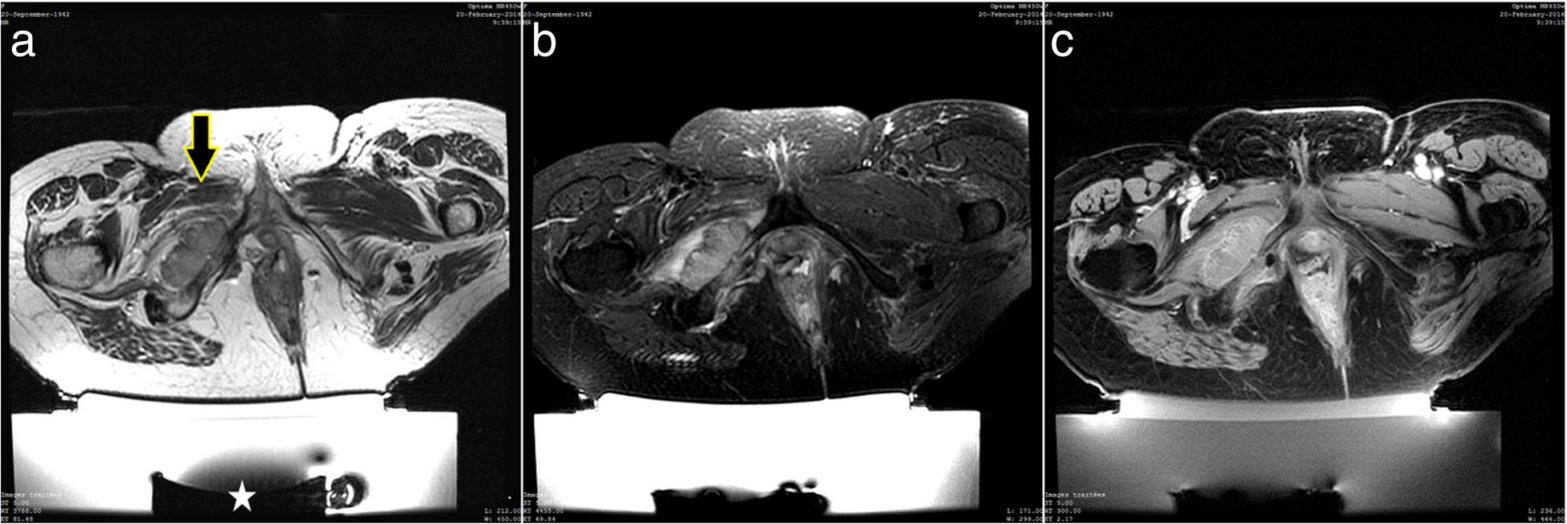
Patient 5. a Axial T2-weighted MR image showing a bone metastasis of the pubic ilio-branch invading adjacent soft tissues (arrow) in front of the transducer (star) before the procedure; b, c. Axial T2-weighted fat-suppressed and T1-weighted fat-suppressed with contrast MR images showing partial destruction of the lesion after the procedure
